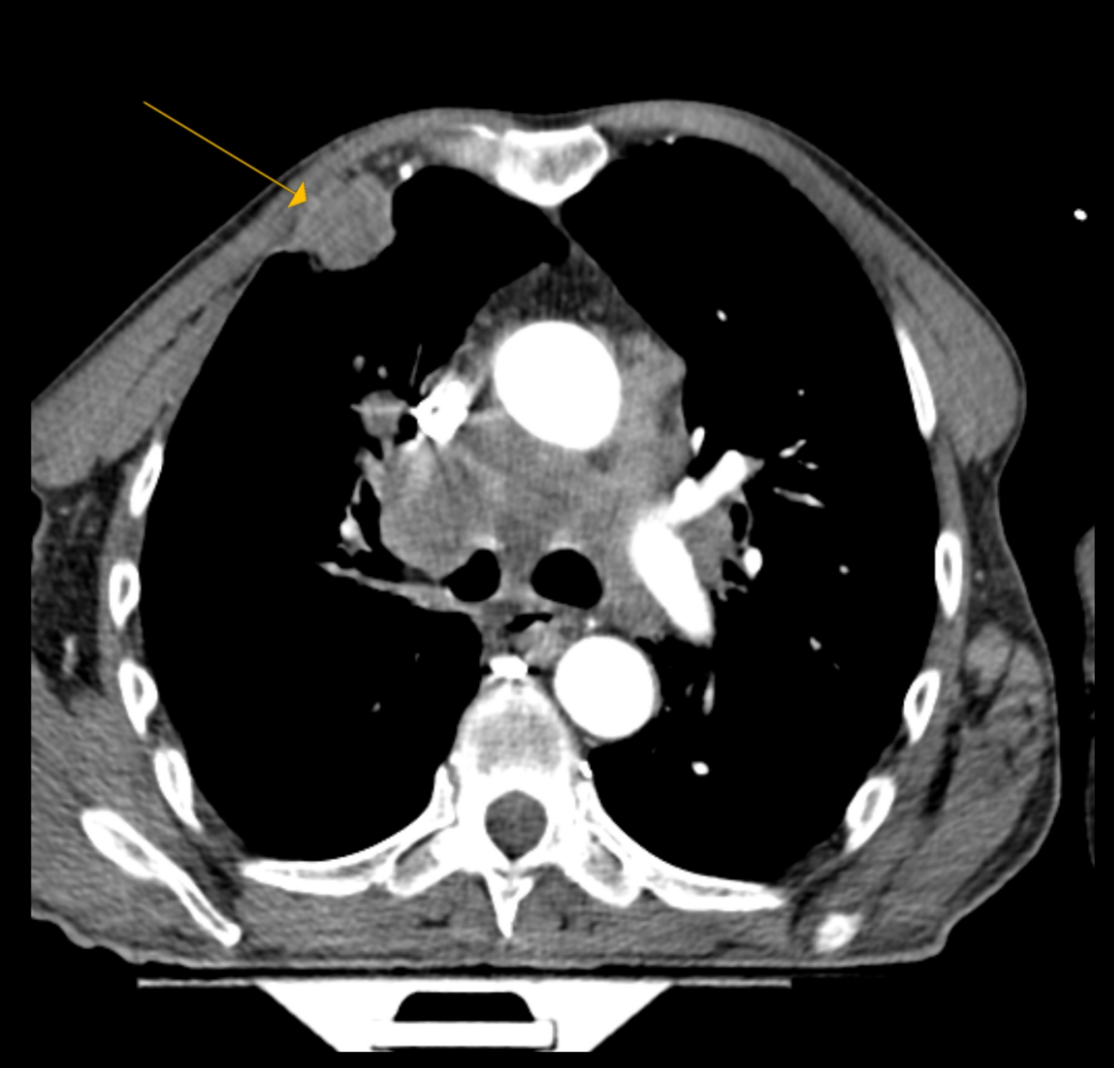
A CT pulmonary angiogram showing a 3.6-cm speculated mass within the anterior right upper lobe CT: computed tomography
